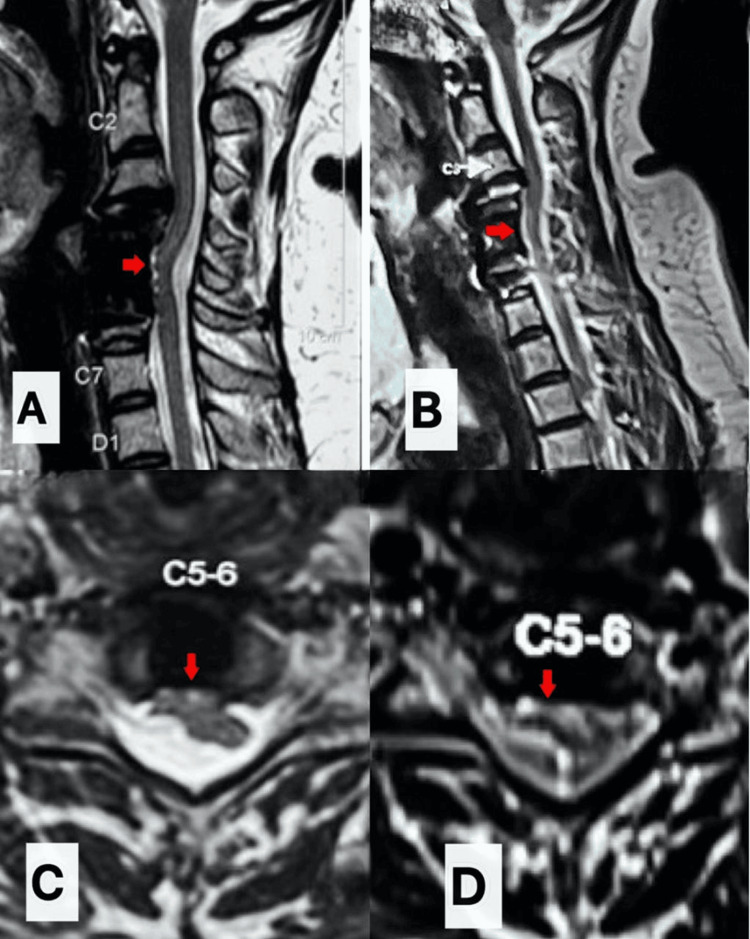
Sequential MRI demonstrating gradual spontaneous restoration of the ventral cerebrospinal fluid (CSF) column. (A) At three months (sagittal), a thin ventral CSF cleft was observed (arrow). (B) At nine months, there was an interval increase in the ventral CSF space, indicating progressive release of anterior tethering. (C, D) Corresponding axial images at three and nine months demonstrate increasing ventral CSF around the cord. These findings suggest gradual spontaneous resolution of ventral cord displacement. MRI: magnetic resonance imaging
